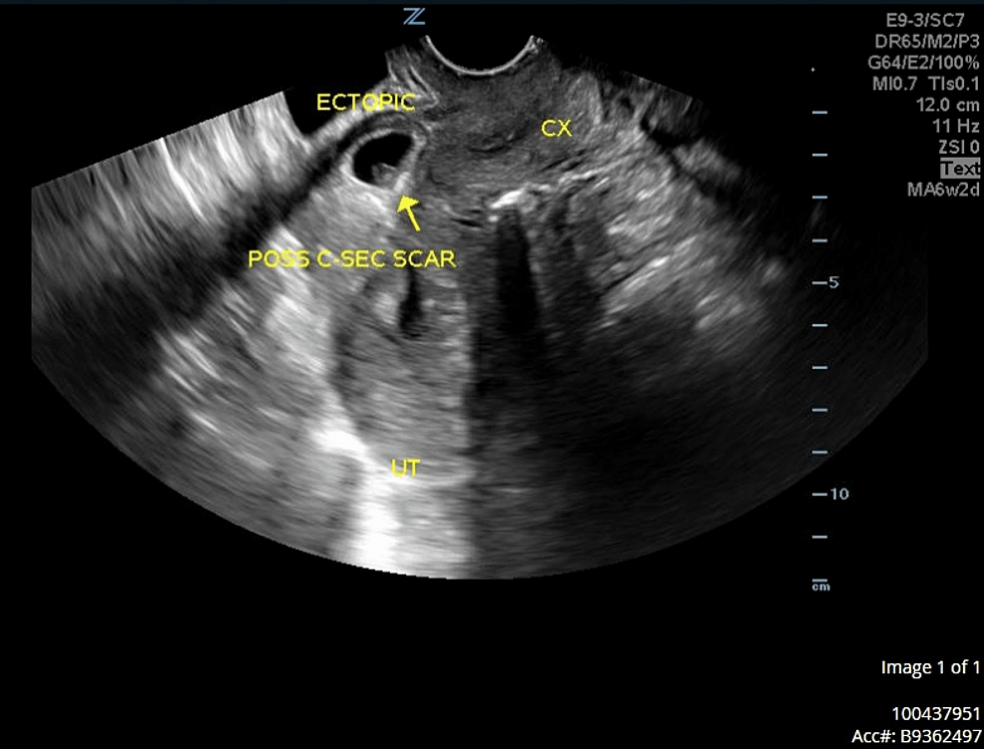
Transvaginal ultrasound (TVUS) (sagittal view) showing fetal pole at the level of the previous CS scar Cx: cervix; UT: uterus
